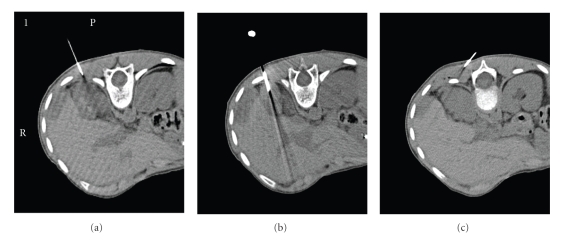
Radio frequency ablation and cryoablation procedure*. Under direct CT fluoroscopic guidance, an RFA electrode was advanced
